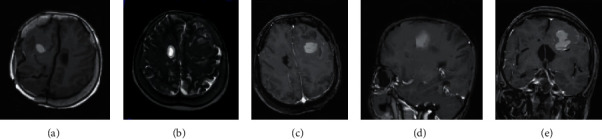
MRI images of multiple intracranial metastatic melanomas.
